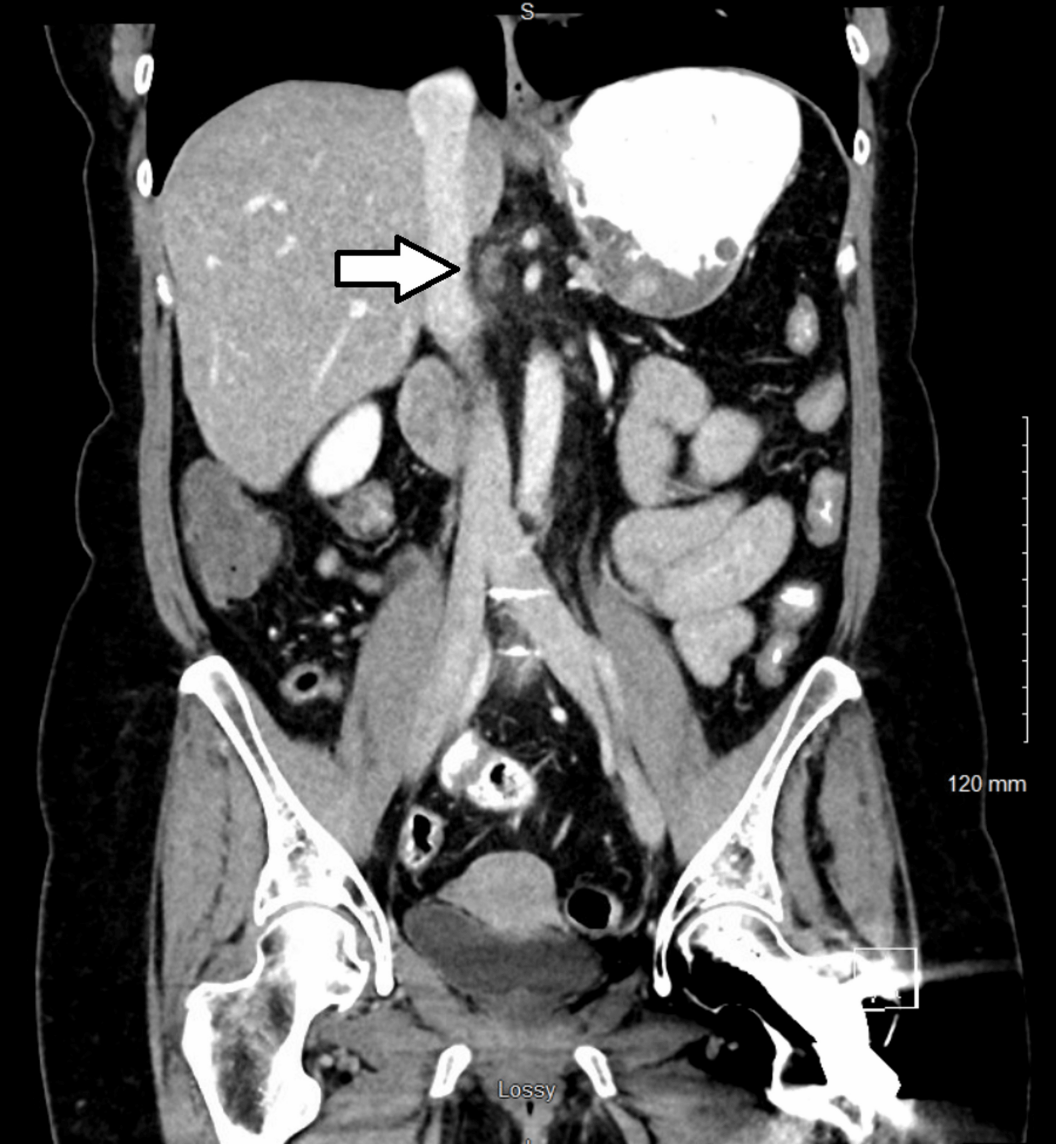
The CT showing one single growing portocaval lymph node (white arrow) CT: computed tomography
